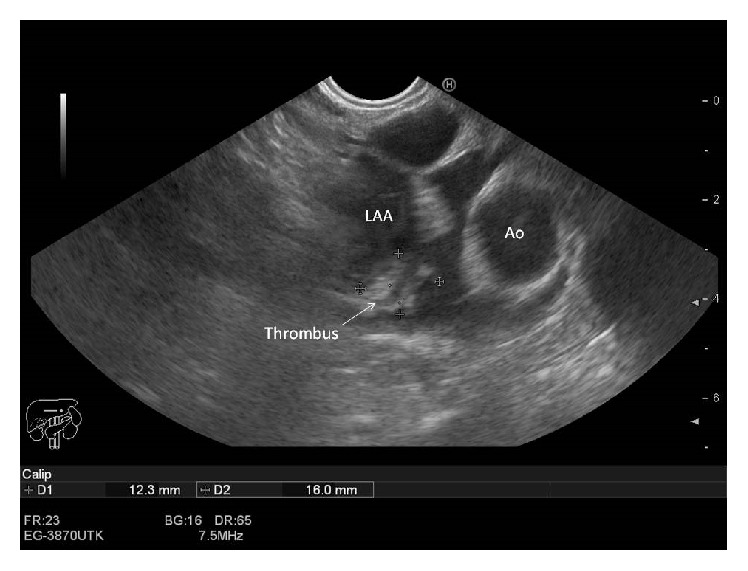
Thrombus detection in the left atrial appendage using endoscopic ultrasound technique. LAA: left atrial appendage; Ao: aortic root.
